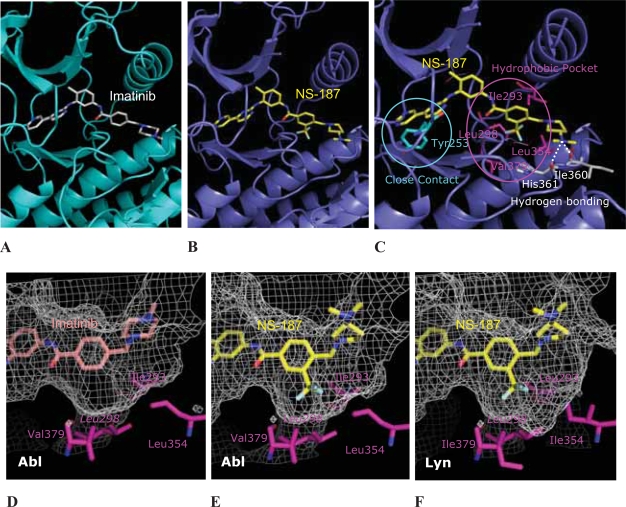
NS-187 and imatinib bound to Abl and Lyn. (A) and (B) are X-ray structures of imatinib/Abl and NS-187/Abl complexes, respectively. (C) shows important interactions between NS-187 and Abl. Comparison of the CF_3_ binding pocket in Abl and Lyn is shown in D–F. Meshes show the molecular surfaces of the kinases. The hydrophobic amino acids forming the hydrophobic pocket are shown in magenta. (D), (E) and (F) show the imatinib/Abl, NS-187/Abl, and NS-187/Lyn complexes, respectively. Adapted from Asaki et al. 2006 and Horio et al. 2007.
